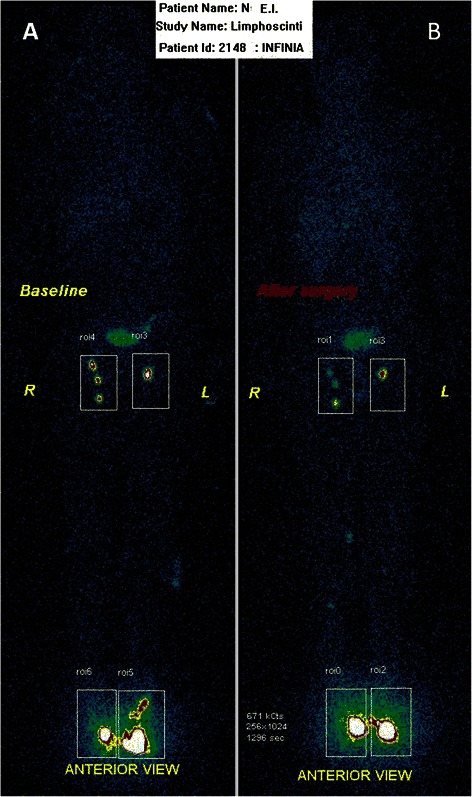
Lymphoscintigrams before the surgery (а) and on Day 7 after the endoscopic vein harvesting on the right lower limb (b)
